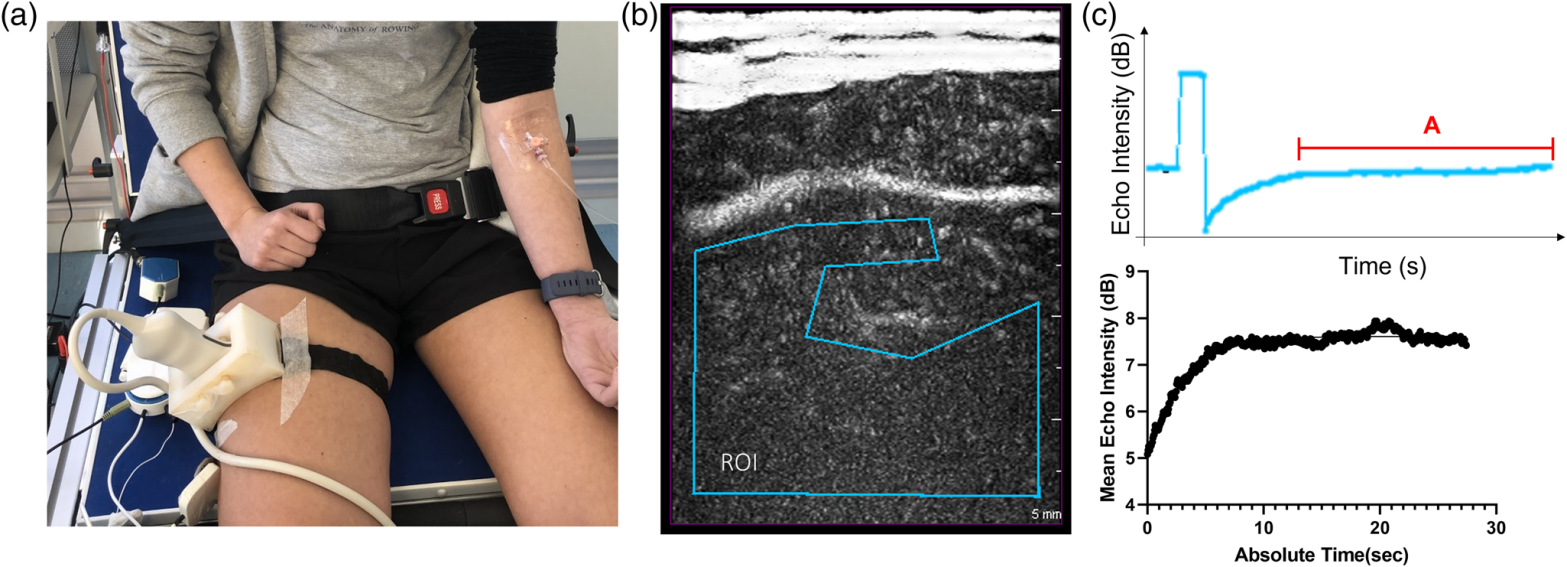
(a) Participant setup for the contrast enhanced ultrasound (CEUS) recordings. (b) A representative image showing a selected region of interest (ROI) avoiding rapid filling vessels. (c) Representative replenishment curve showing the *A* value (microvascular blood volume) from the plateau and one phase linear association to calculate *A* and β values.
